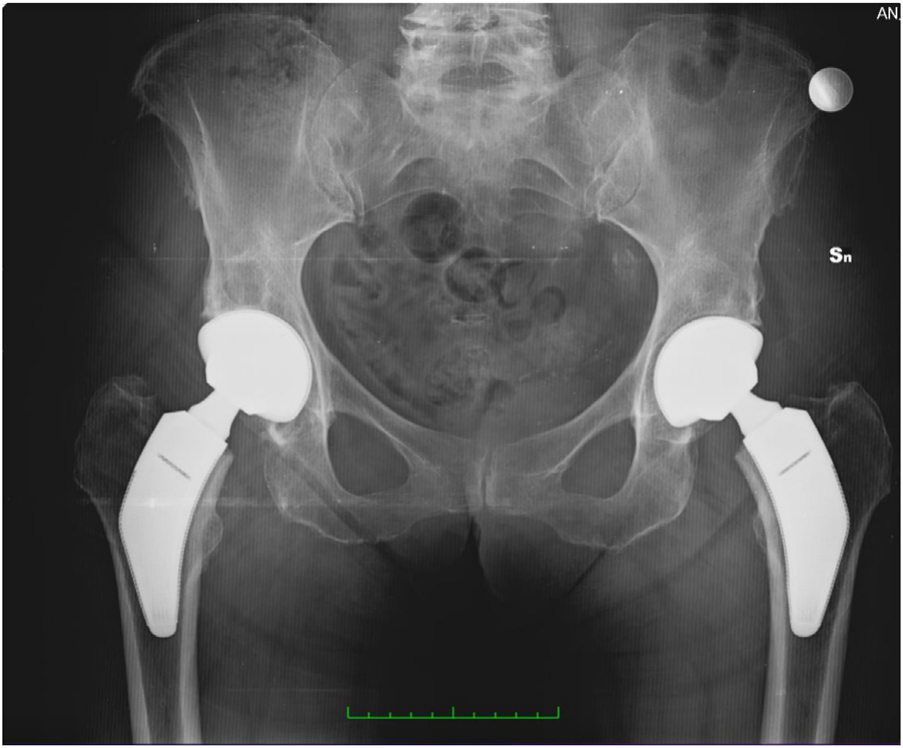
Six-year follow-up x-ray of a bilateral total hip arthroplasty.
